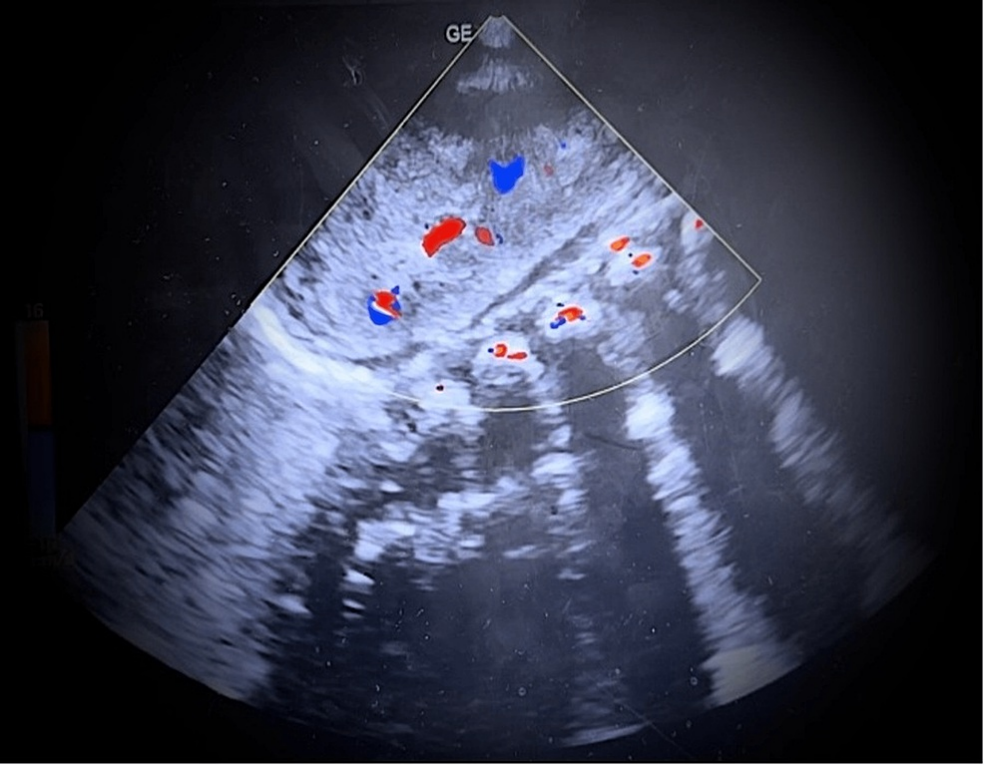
Color Doppler image of the left kidney showing the “red, white, blue” sign (artery, pyramid, vein).
